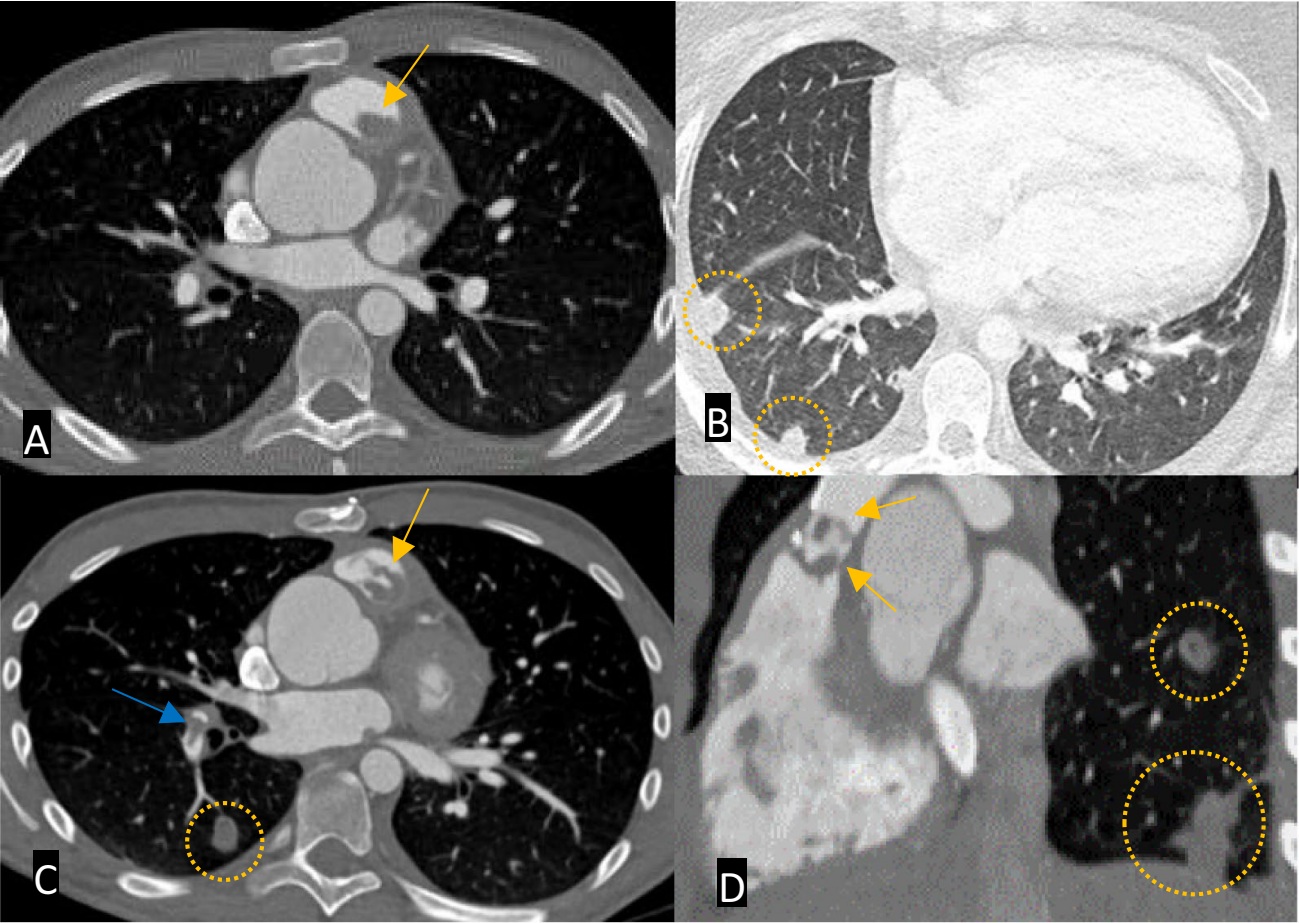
Select multiplanar CT images showing a valved RV-PA conduit to treat RVOT obstruction. Axial images in the bone window (A and C), lung window (B) and MPR reformats in the sagittal plane (D), bone window. There are vegetations of the RV-PA conduit (orange arrows), septic emboli in the arteries of the right lower lobe (blue arrow) and pulmonary abscesses (dotted circles). RV: right ventricle; PA: pulmonary artery; MPR: multiplanar reconstruction; RVOT: right ventricular outflow tract
